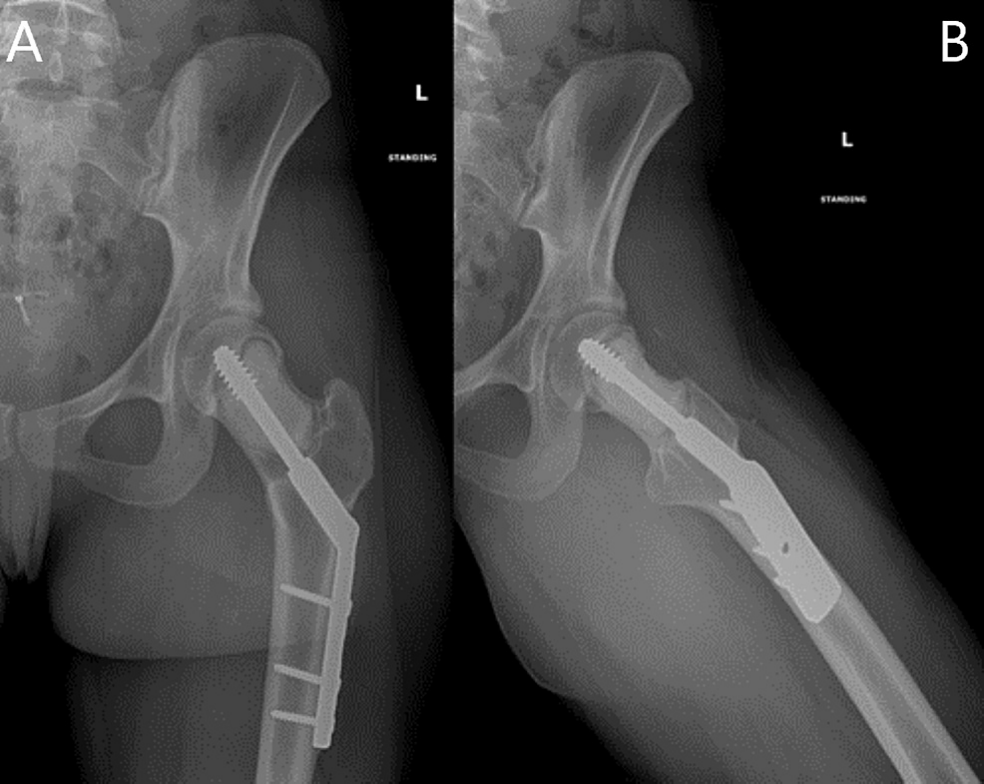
Radiographic anteroposterior (A) and frog-leg lateral (B) views of the left hip taken at one-year follow up reveal no areas of osteolysis
